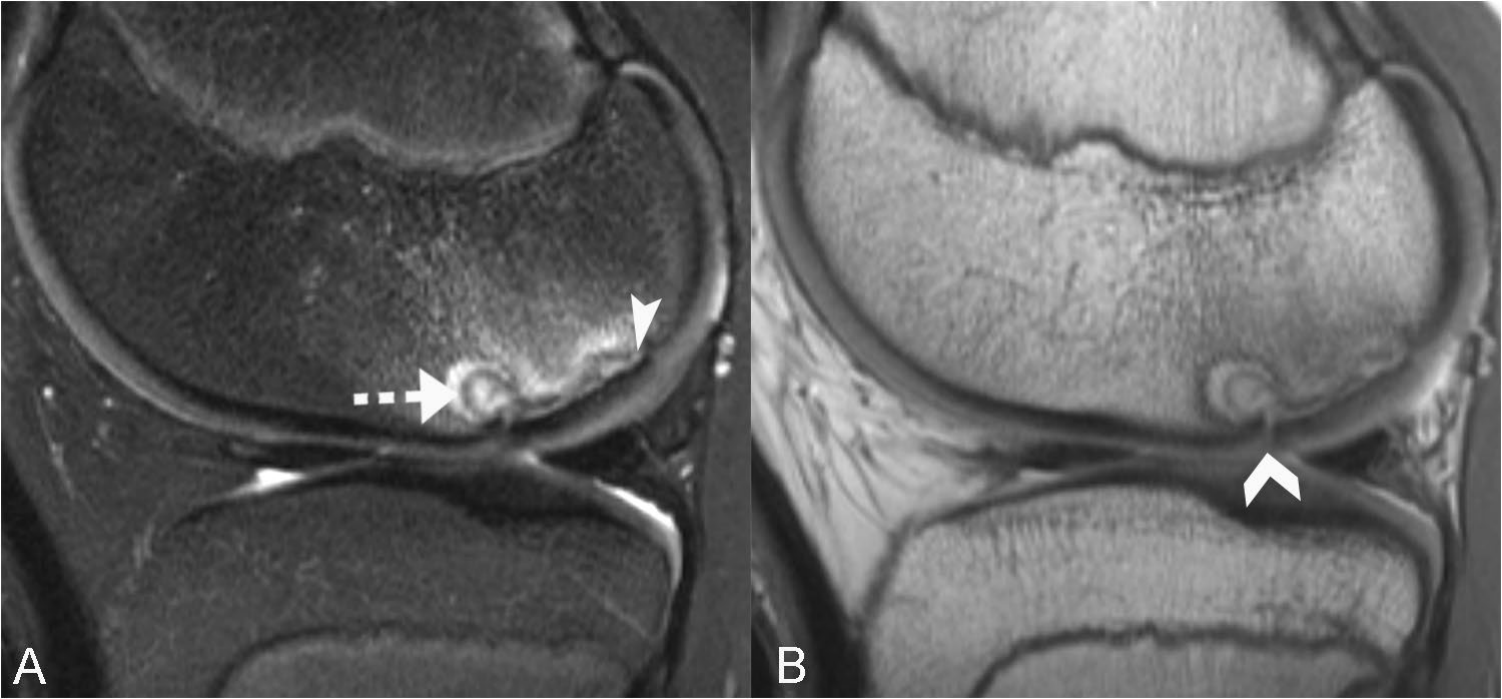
Unstable LFC OCD lesion in a 15-year-old skeletally immature male. Sagittal T2-weighted fat-suppressed (A) and intermediate-weighted (B) MR images show an intermediate signal intensity progeny-parent bone interface (arrowhead, A) that is defined by the underlying parent bone marginal rim. At the anterior margin of the OCD lesion, there is a 4.5-mm cyst (dashed arrow) that underlies a hyperintense cartilage fissure (chevron). A ballotable unstable OCD lesion was confirmed at arthroscopy.
